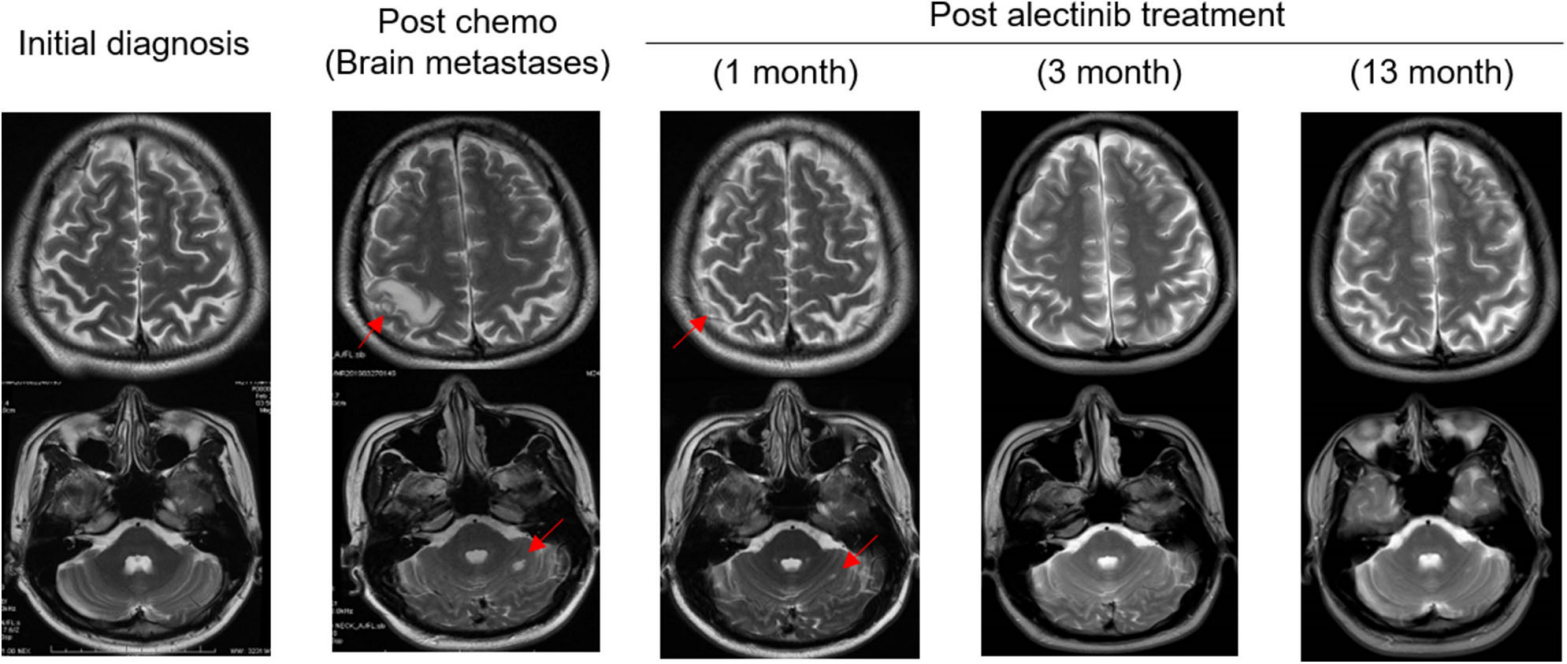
Serial MRI scans showing patient's metastatic brain tumors responded completely to alectinib therapy. The patient achieved partial response (PR) 1 month after the initiation of alectinib therapy, and the lesions disappeared (CR) completely at the 3- and 13- month post-alectinib treatment time points. Arrows: metastatic brain tumors. CR, complete response.
